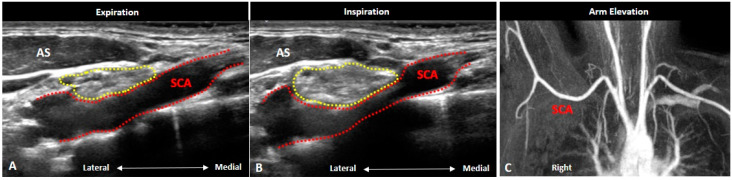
Ultrasound imaging of thoracic outlet syndrome caused by subclavian artery compression in a young woman suffering right arm pain during inspiration. Ultrasound images during expiration (A) and inspiration (B) showed sudden narrowing of the subclavian artery during inspiration (red dashed area). Focal stenosis of the subclavian artery was not obvious in the magnetic resonance angiography during right arm elevation (C). Inferior trunk: yellow dashed area. AS: anterior scalene muscle; SCA: subclavian artery.
